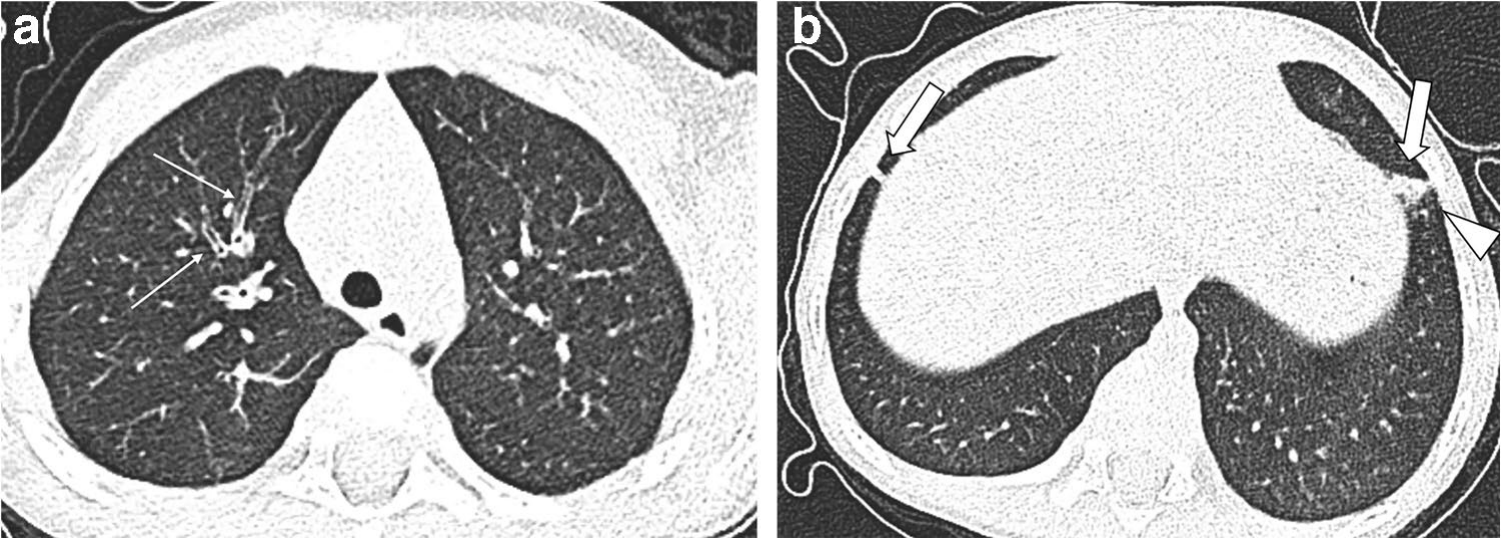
Acute pulmonary exacerbation in a 3-year-old boy. a, b Upper lobe (a) and lower lobe (b) sections of axial free-breathing CT without sedation show clear bronchial wall thickening (*thin arrows*) and focal parenchymal abnormalities in the lower lobes (*thick arrows*). The consolidation in the left lower lobe also shows a ground-glass halo (*arrowhead*), making it suspicious for acute respiratory infection
